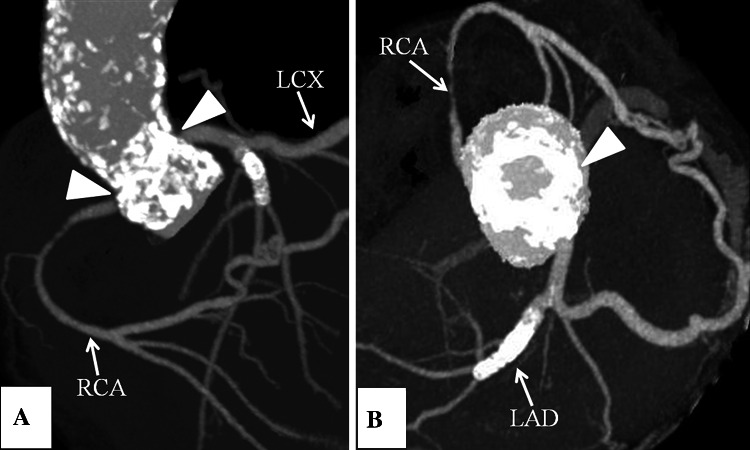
Computed tomography images showing severely calcified aortic root. A calcified plate at the STJ (*arrow heads*) made supravalvular aortic stenosis. a Long axis view, b Short axis view. *LAD* left anterior descending coronary artery, *LCX* left circumflex coronary artery, *RCA* right coronary artery
